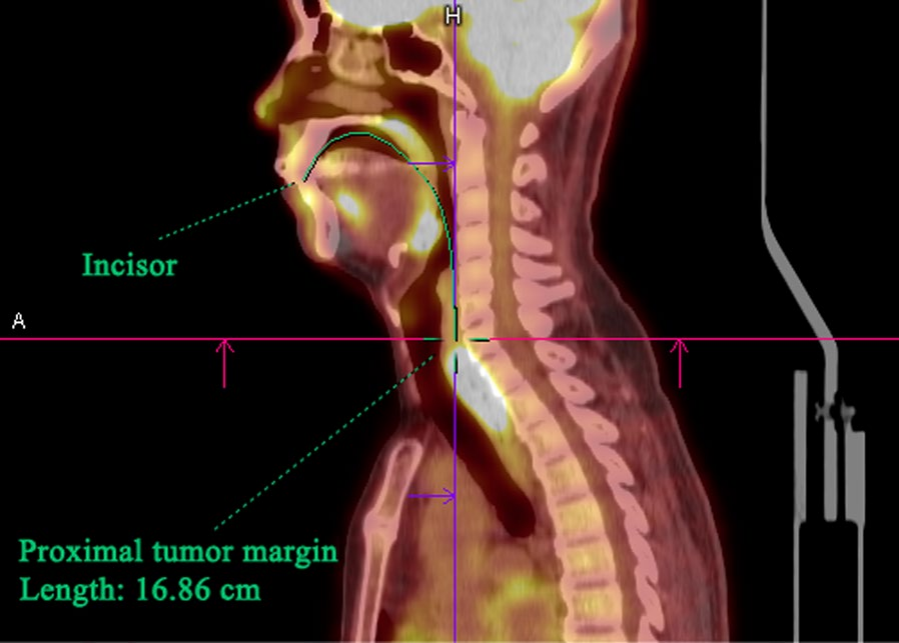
By mimicking the pathway of the endoscope, a polyline (green) was drawn from the patient’s incisor along the oral cavity, inferior margin of the palate, pharynx, and esophagus to the proximal esophageal tumor margin on the fused sagittal PET/CT image
